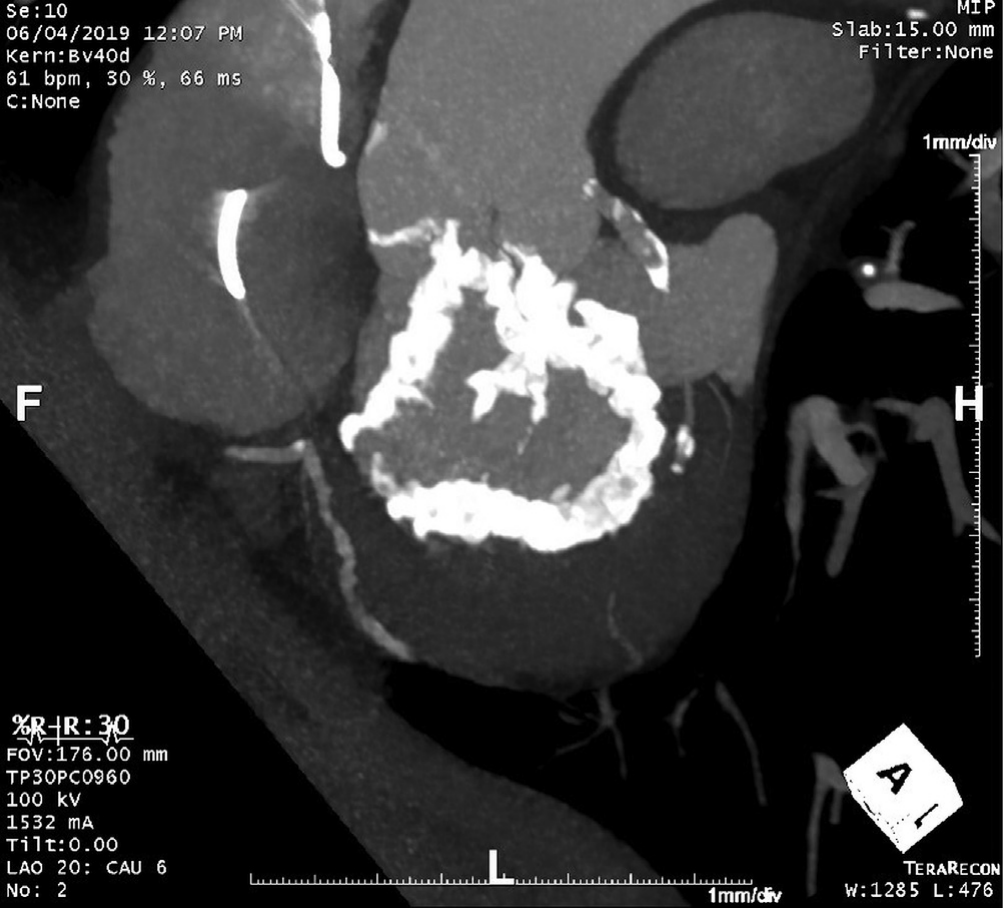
Computed tomography heart demonstrating severe circumferential mitral annular calcification extending into aortic annulus.
